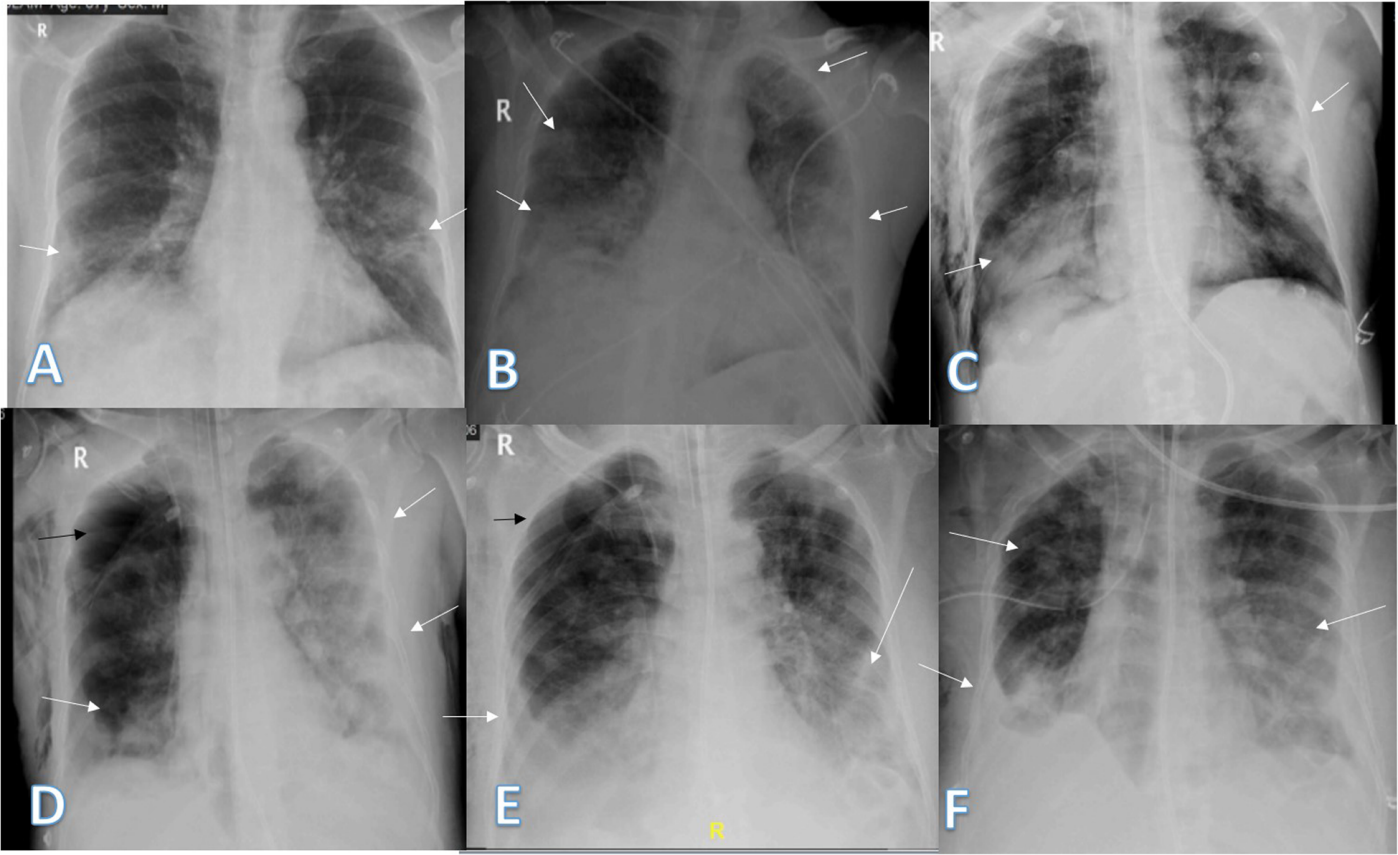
67-year male with positive COVID-19 virus. Initial X-ray (a) showed small right and left lower zonal midzonal air space consolidation opacities (arrows) with TSS as 2. On the 3rd day (b), air space consolidation opacities increased extended to involve most of the left lung and the lower zone of the right lung with midzonal patches (arrows); TSS reached 5 (3 on LT side and 2 on right side). The patient deteriorated and was hypoxemic, so he was intubated. On the 7th day (c), the patient developed pneumothorax and ICT was inserted. On the 9th day (d), 13th day (e), and 16th day (f), there was air space consolidation (arrows) more on the left lung with mild pneumothorax (black arrows on d and e). Minimal right pleural effusion was seen on (short arrows on e and f). The total severity score was 6 on d–f; the patient died
